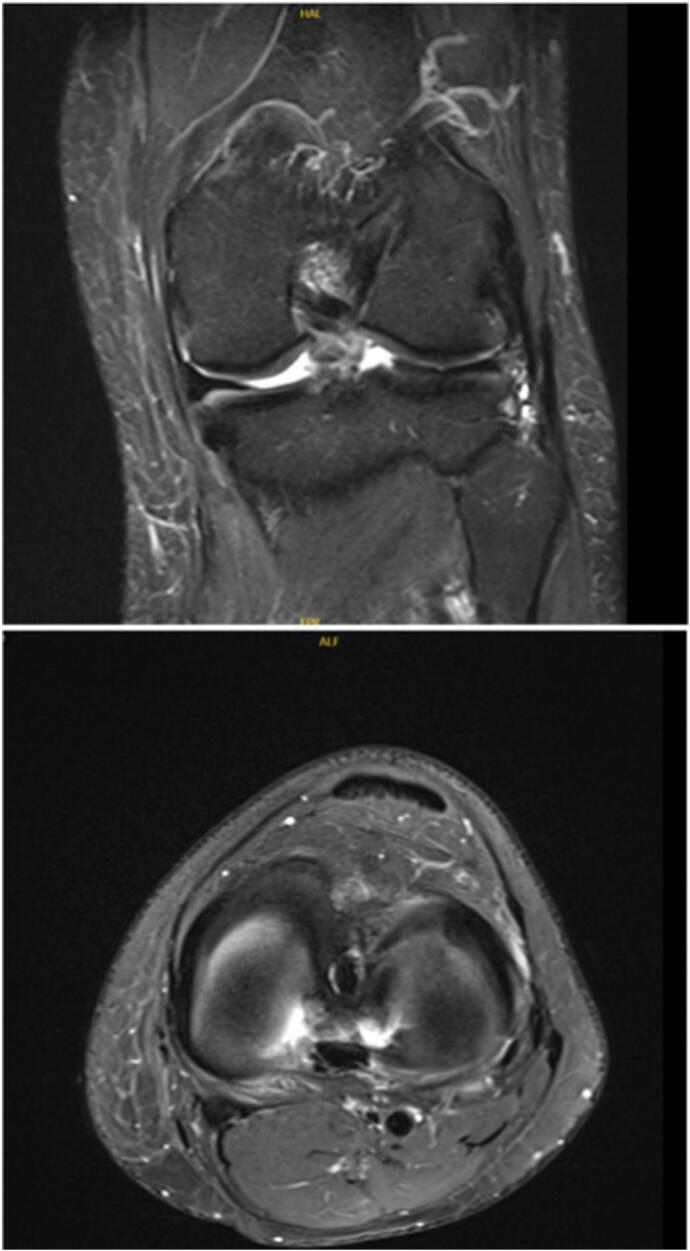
MRI (coronal and axial cuts) showing fraying and loss of meniscal tissue at the posterior horn of the medial and lateral meniscus.
